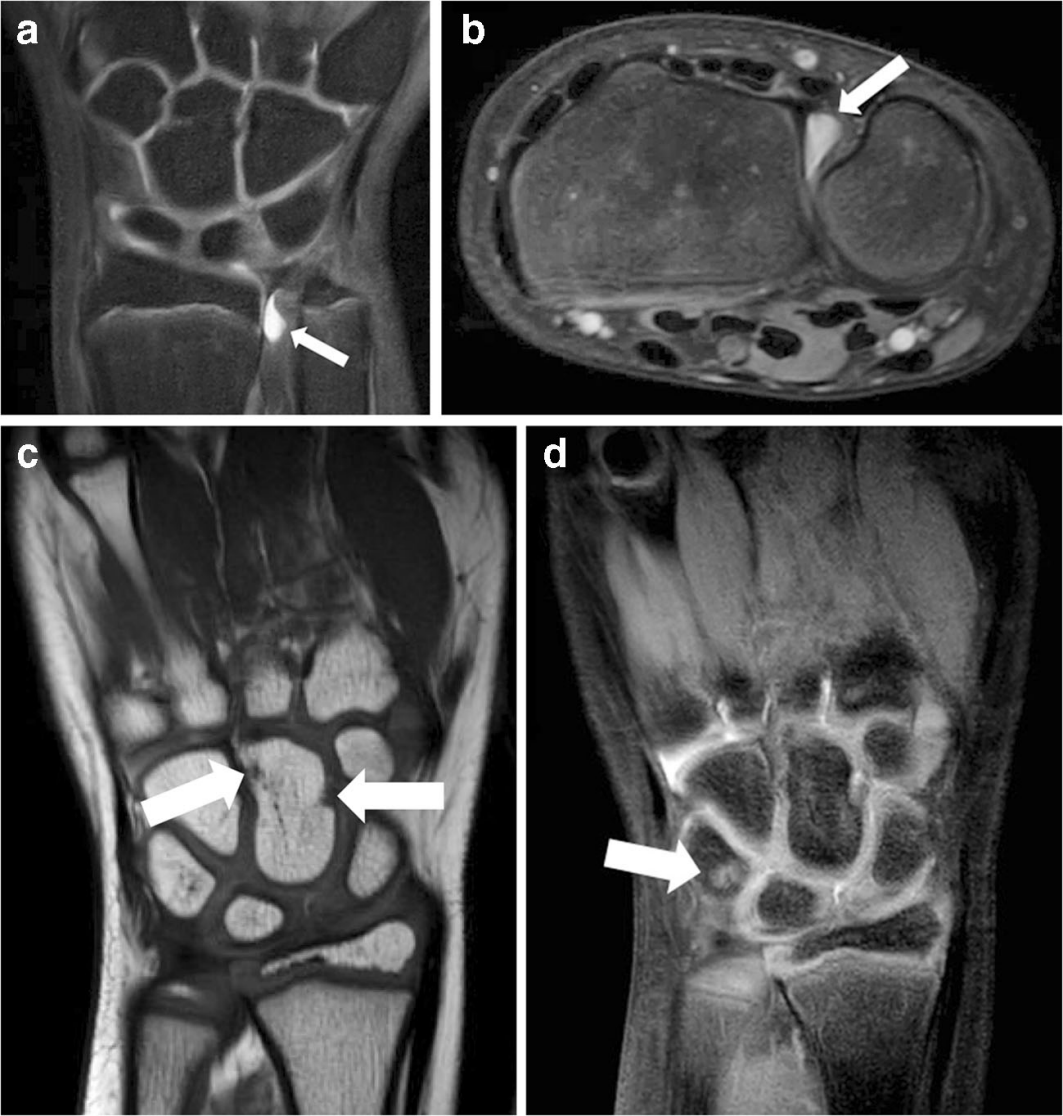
Examples of joint fluid, bony depressions and medullary changes suggestive of bone marrow edema. a, b Coronal (a) and axial (b) T2-weighted fat-saturated images in a 13-year-old girl show joint fluid (*arrows*) in the distal radioulnar joint. c Coronal T1-weighted mDixon in-phase image in a 7-year-old girl shows bony depressions (*arrows*) in the capitate. d Coronal single-point Dixon (sense: water) image in a 7-year-old girl shows signal changes suggestive
